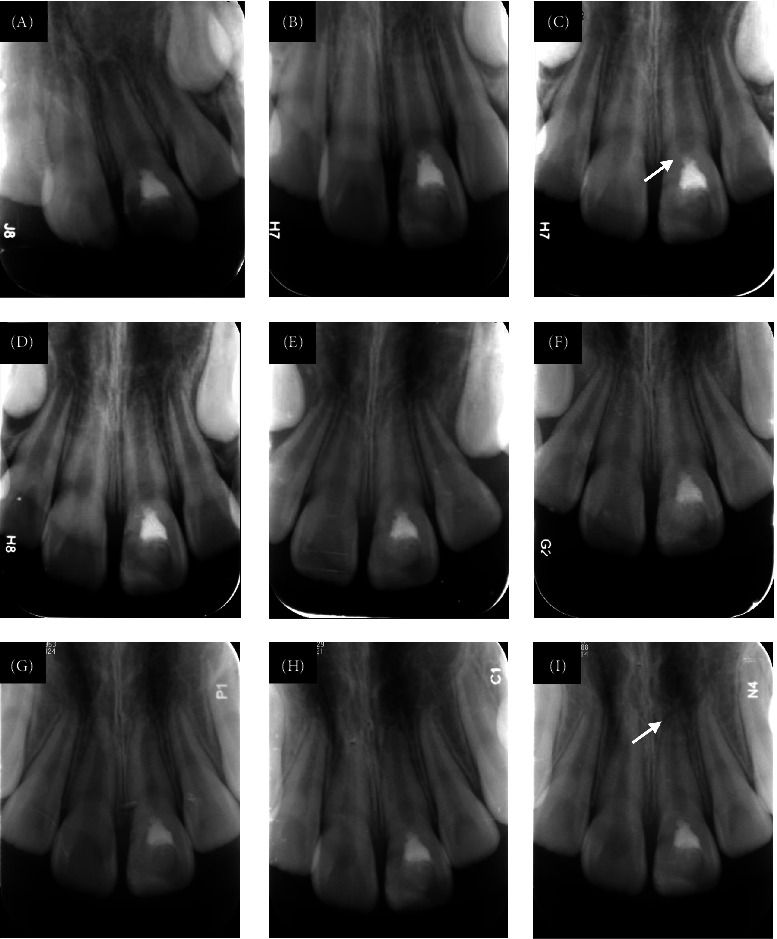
Periapical radiographs of tooth 21 of a 7-year-old girl with a complicated crown fracture and immature root formation that underwent full pulpotomy. (A) Immediate postoperative radiograph. (B) Follow-up radiograph at 1 month. (C) Follow-up radiograph at 3 months, white arrow points to the dentin bridge. (D–H) Follow-up radiographs at 6, 12, 18, 24, and 30 months. (I) Follow-up radiograph at 44 months showed that the apical foramen was closed (white arrow).
